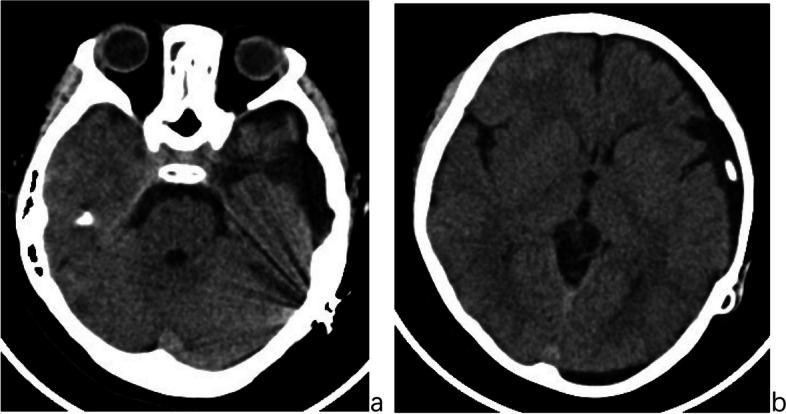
a and b Cranial CT scans obtained 5 years after the subdural-peritoneal shunt, demonstrating a decrease in both the arachnoid cyst and subdural effusion
